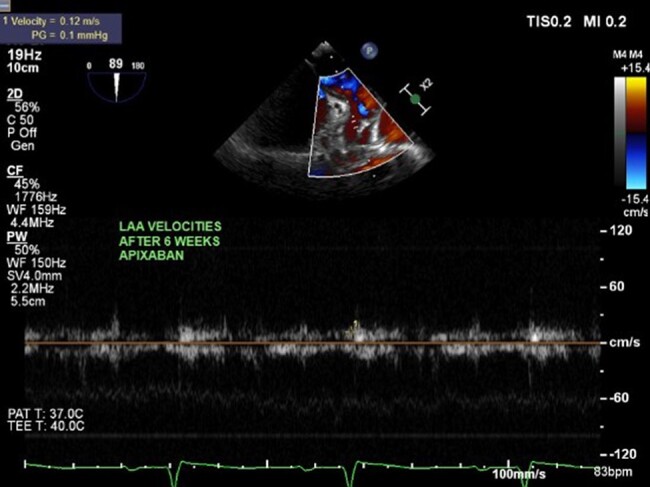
Low emptying left atrial appendage velocities (8 cm/s: *N* 60 ± 14 cm/s) reveals persistent hypokinetic left atrial appendage (pulsed Doppler evaluation of left atrial appendage during transoesophageal echocardiogram).
